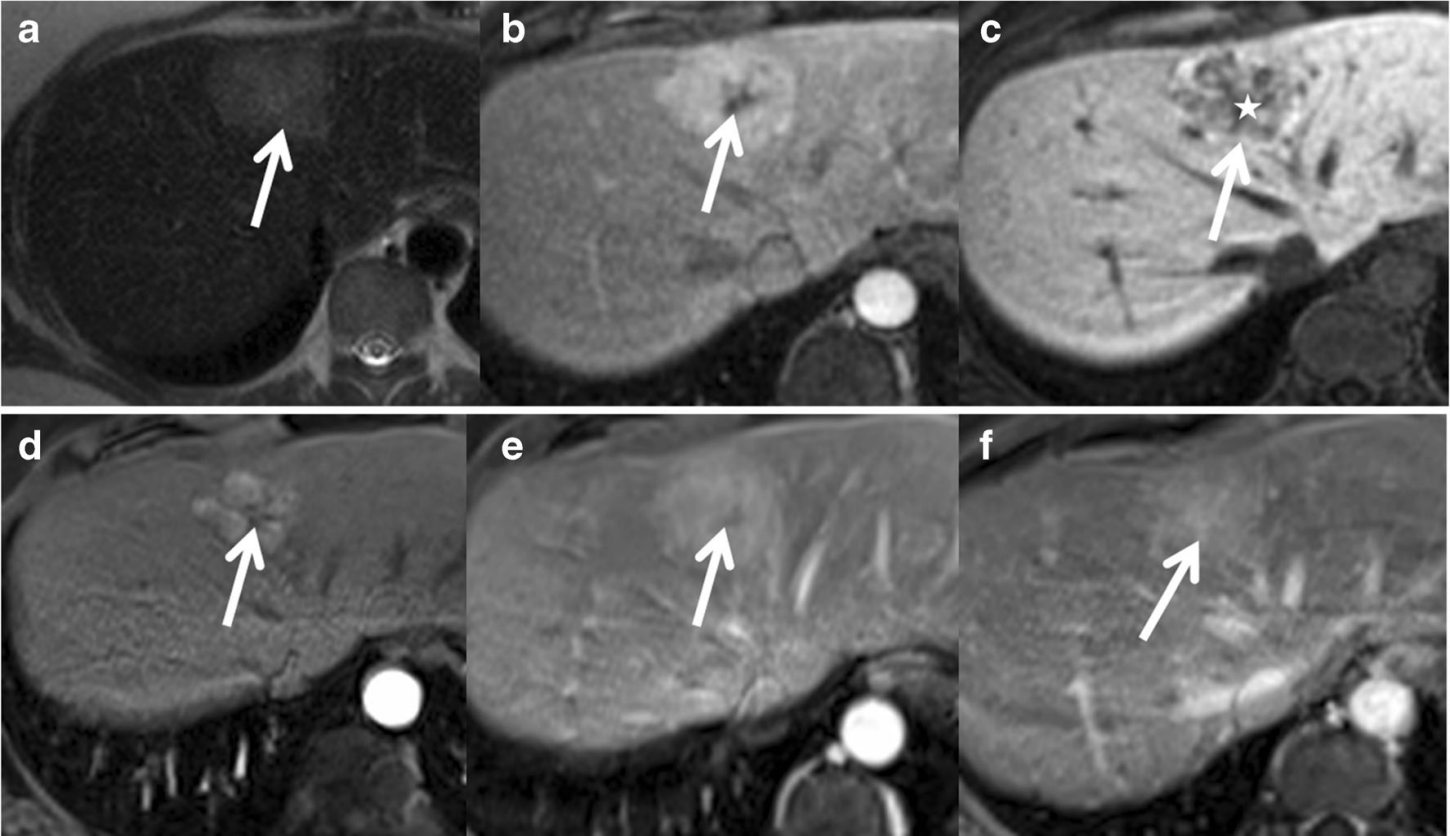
Appearance of FNH with hepatobiliary-specific contrast (gadoxetate) and a routine extracellular agent (gadolinium). (a) Axial T2W MRI shows iso- to mildly hyperintense lesions in segment 4 of the left lobe of the liver (*arrow*). (b) Axial post-contrast MRI with gadoxetate shows intense enhancement of the lesions in the arterial phase with a non-enhancing central scar (*arrow*). (c) Axial post-contrast MRI in the 20-min hepatobiliary phase shows a peripheral ring of hyperintensity (*arrow*) with central non-enhancement (asterisk). Follow-up MRI with gadolinium after 6 months shows an enhancing lesion with a non-enhancing scar (*arrow*) in the arterial phase (d) and persistent enhancement in the portal venous (e) and delayed phases (f) with delayed enhancement of the central scar (*arrow*)
